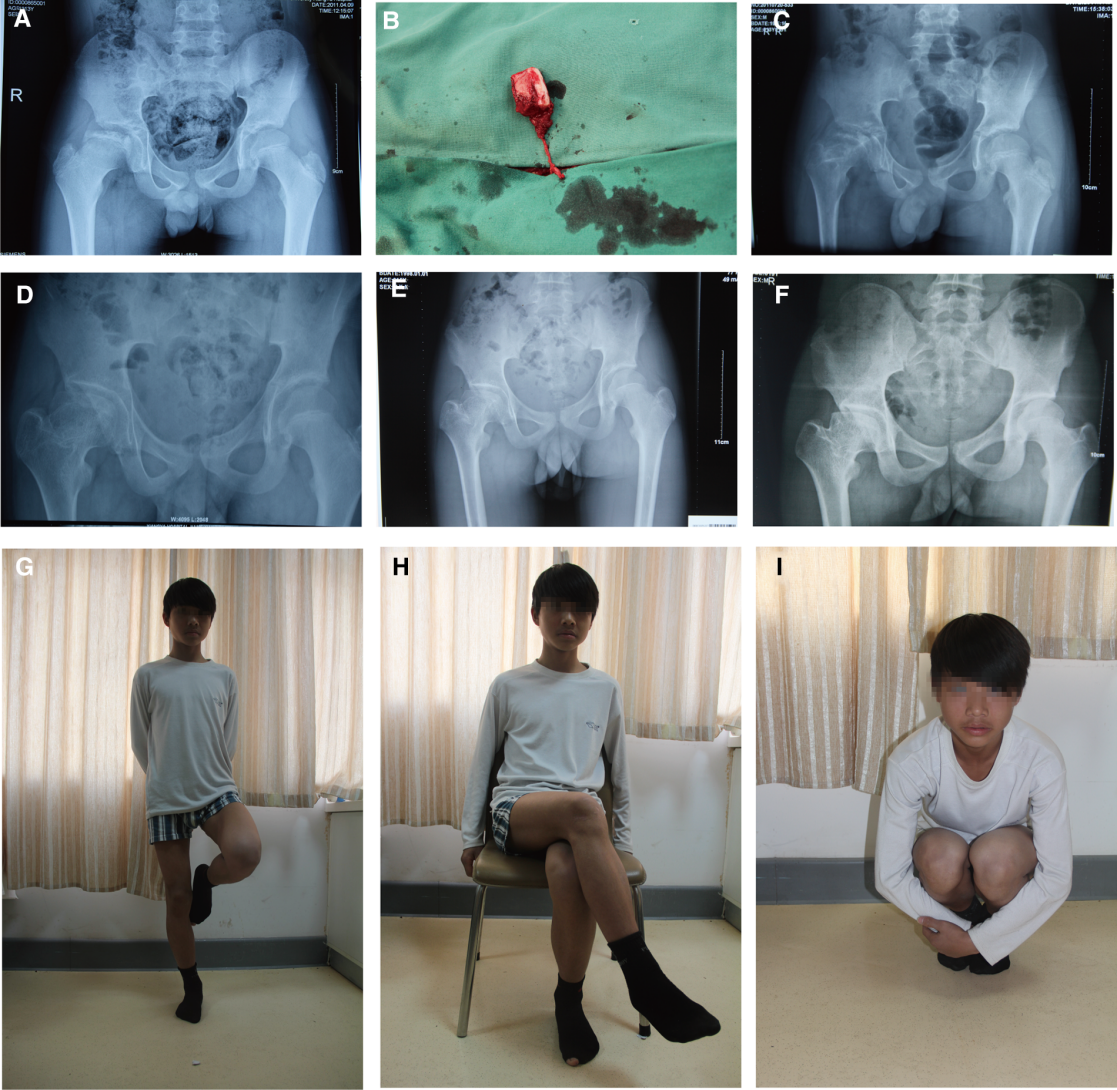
A 13-year-old boy underwent a pedicled iliac bone flap based on deep circumflex iliac vessels to treat LCPD of the right side (lateral pillar grade B/C). (A) Preoperative x-ray film. (B) The pedicled iliac bone flap was harvested. Postoperative AP x-rays at (C) 3 months, (D) 17 months, (E) 24 months, and (F) 69 months showed no collapse of femoral heads or narrowing of the hip joint spaces. Hip (G) extension, (H) external rotation, and (I) flexion showed good recovery. LCPD, Legg–Calvé–Perthes disease; AP, anteroposterior.
